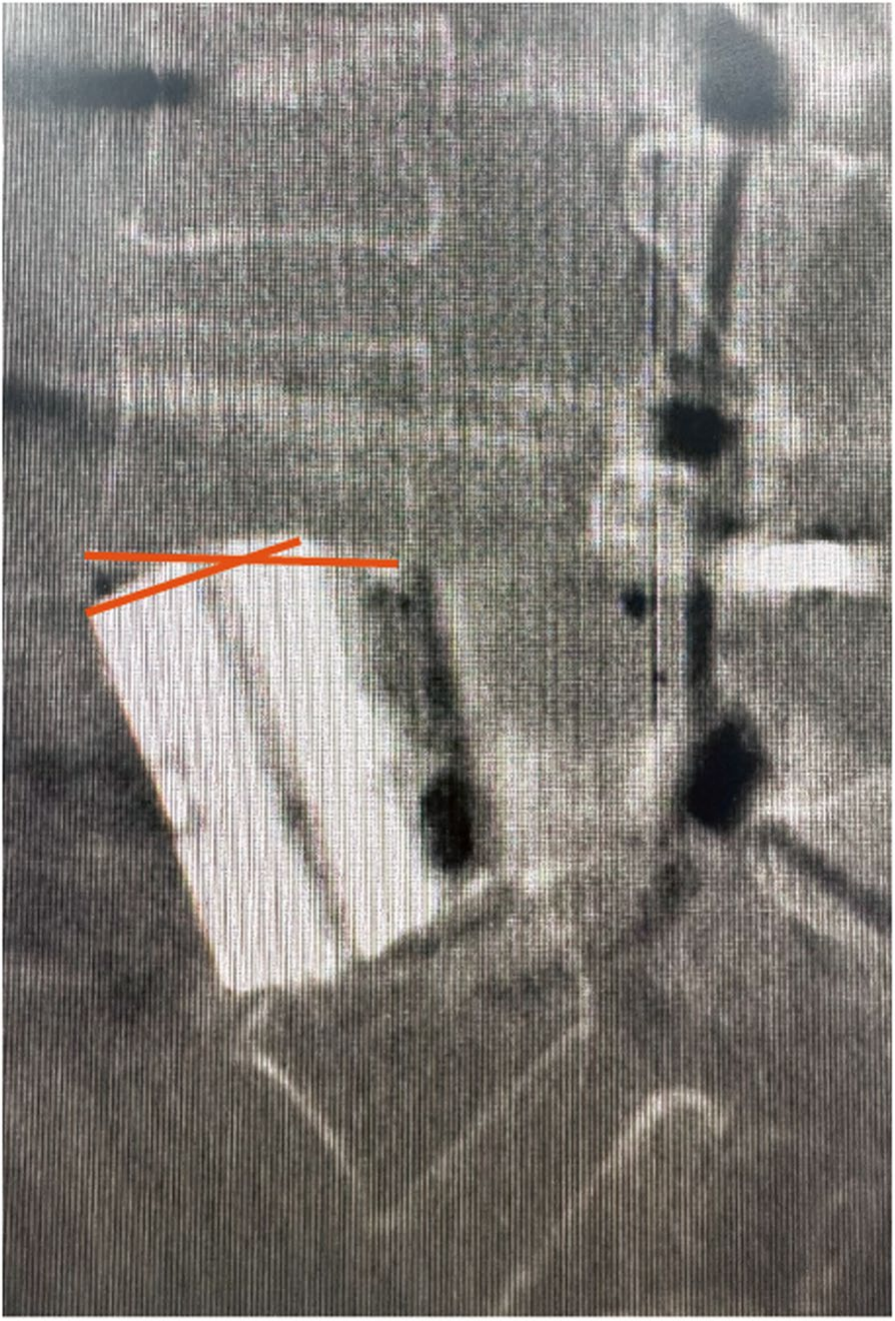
Mismatch of the prosthesis was defined as the angle between the endplate (or osteotomy plane) and the artificial vertebral body exceeding 10° on the immediate postoperative computed tomography scan
